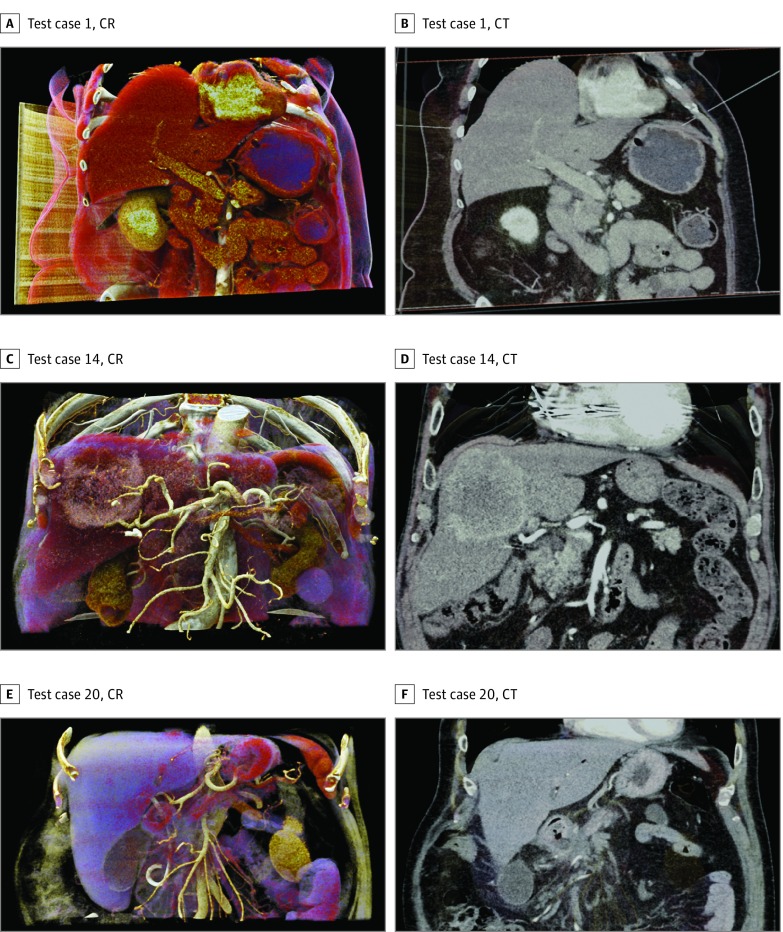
Example Starting Images and Questions From Patient Cases Cinematic rendering (CR) and computed tomography (CT) images. A and B, test case 1 (Does the pancreatic head tumor have contact to the superior mesenteric artery?); C and D, test case 14 (Is the tumor supplied by vessels from the left hepatic artery?); and E and F, test case 20 (Show and name the arterial supply of the left liver lobe).
